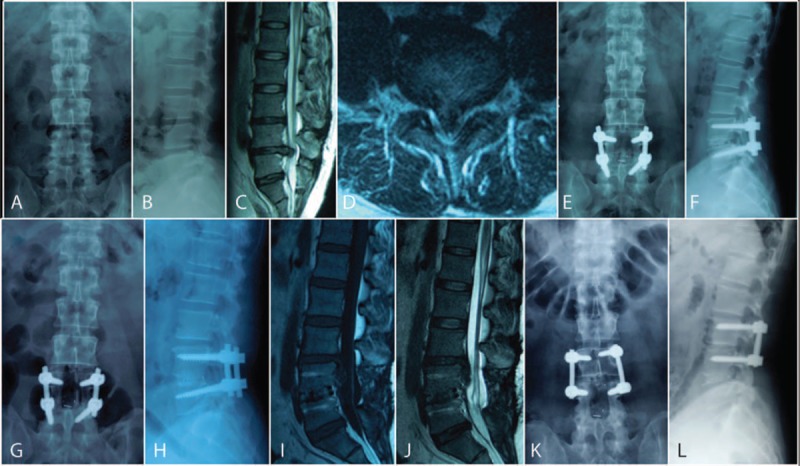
(A–D) Preoperative L_4–5_ disc herniation, and L_3–4_ disc degeneration of Pfirrmann Grade III on MRI. (E and F) L_4–5_ instrumented fusion without intervention to the L_3–4_ disc. (G and H) Bony fusion between cage and endplate in L_4–5_ disc space at 1 year follow up. (I and J) Intervertebral disc prolapse on L_3–4_ 27 months after spinal fusion of L_4–5_. (K and L) Revision surgery of L_5_ pedicle screws taken off and L_3–4_ instrumented fusion was performed.
